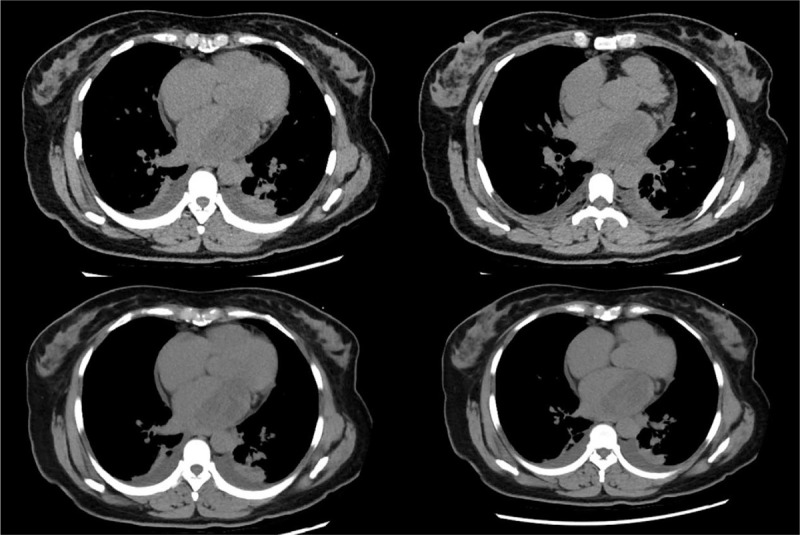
CT scanning showed an increase in the volume of the left atrium; a slightly low-dense patchy mass was visible inside the left atrium, with a CT value of about 25 HU.
